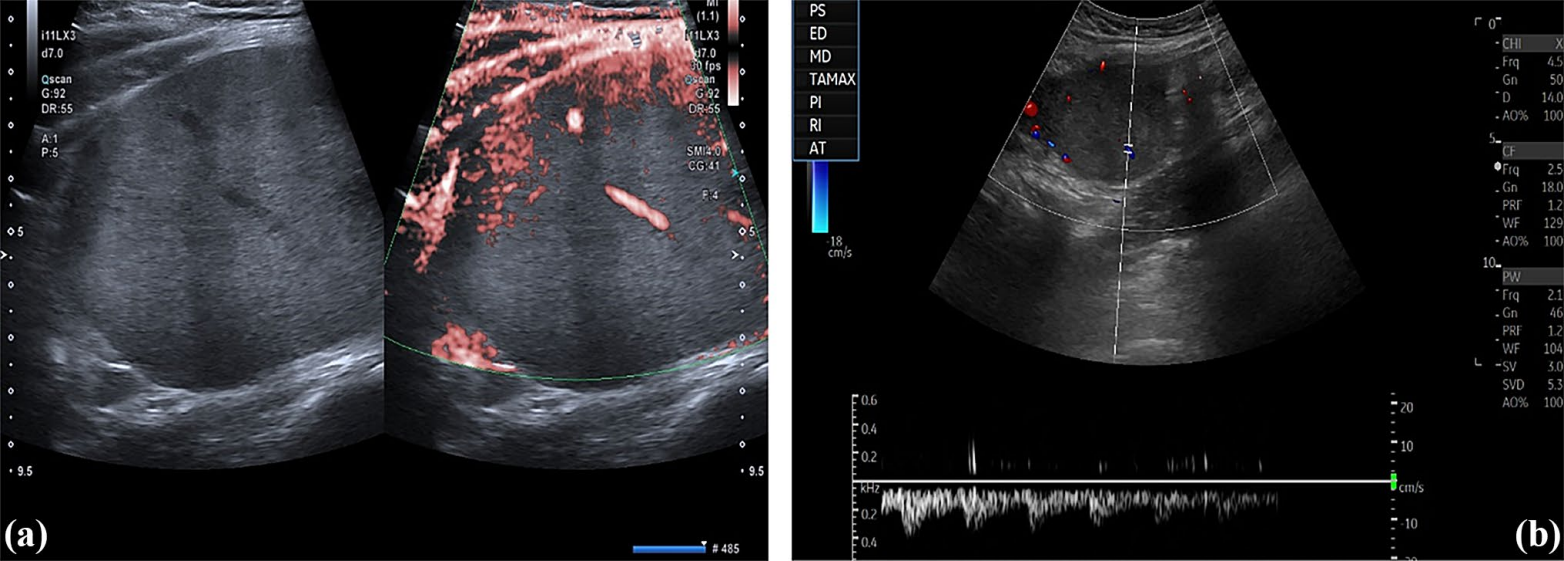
Echoic mass in right lower quadrant with some intralesional vascular signals (a). Color-Doppler detected low-resistance arterial vascular signals (b)
